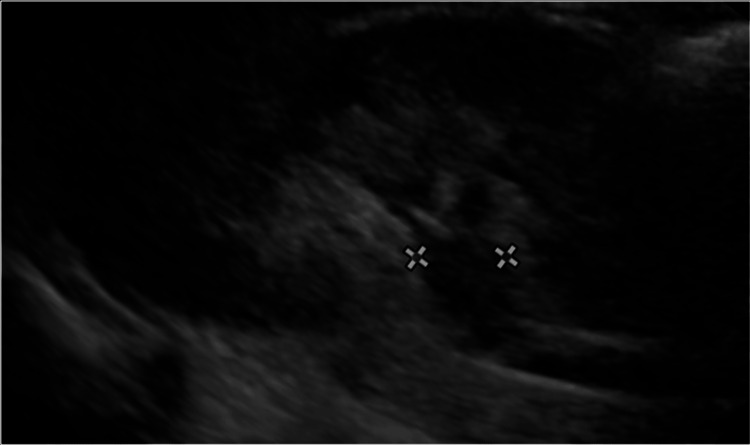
Grayscale ultrasound of the right kidney showing hydronephrosis Grayscale ultrasound image of the right kidney demonstrates a dilated renal pelvis consistent with hydronephrosis. The renal parenchyma appears normal, and no ureteral calculi are visualized.
